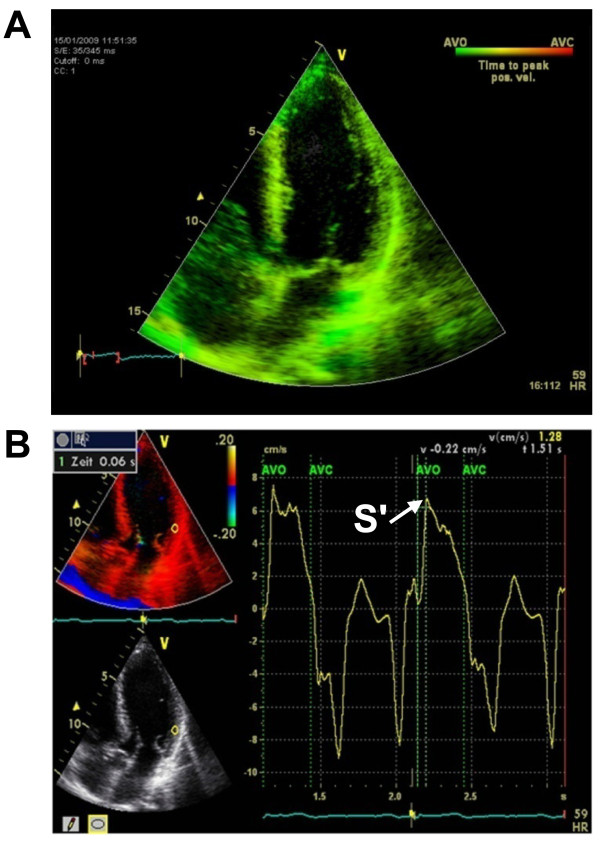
A) TSI in the apical four-chamber view. All LV segments are color-coded in green. Therefore, S' must be within the first third of the transaortal flow in the following TDI measurements. B) TDI in the apical four-chamber view. The interval between the aortic valve opening (AVO) and the peak systolic velocity (S') is 60 ms. In agreement with the TSI data, S' can be found within the first third of the transaortal flow.
